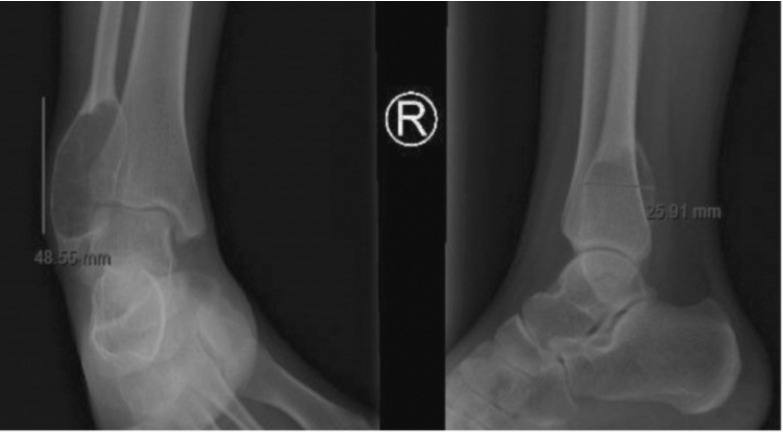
Plain radiograph of the ankle in anteroposterior and lateral views.
